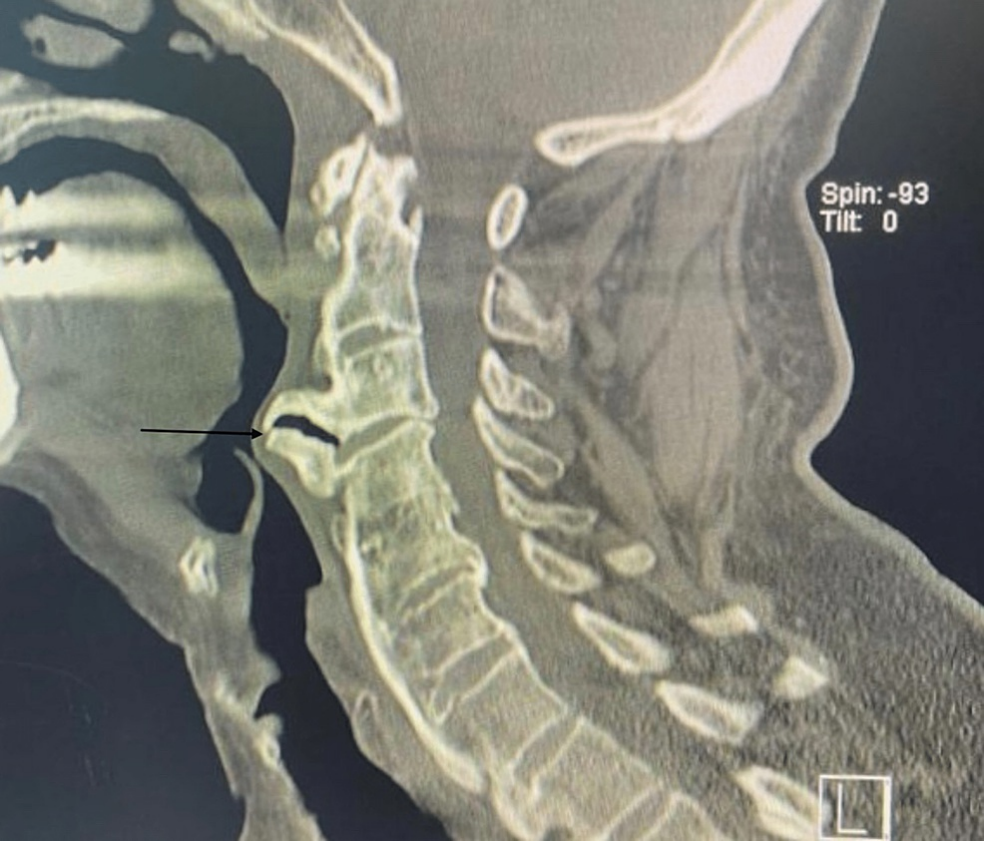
CT scan without contrast; sagittal view Huge anterior osteophytes were observed at the C3-4 level, compressing the esophagus (parrot beak sign)
